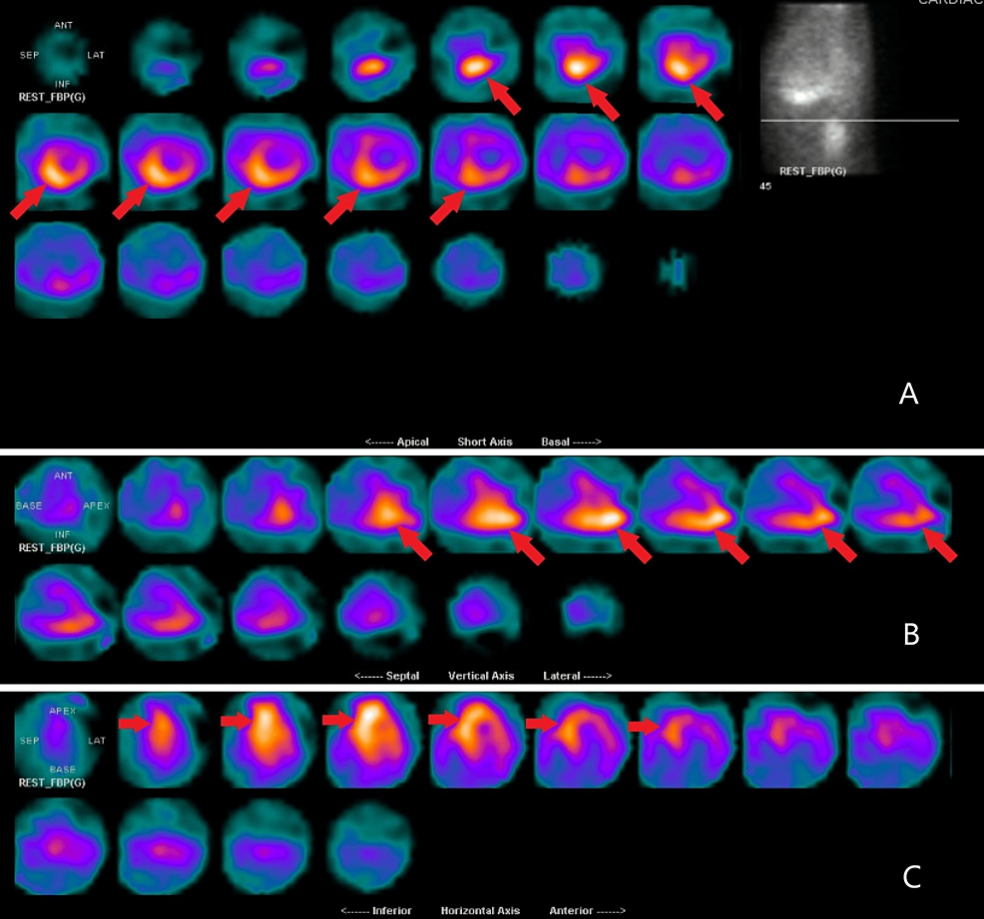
Supine SPECT/CT 99mTc-PYP imaging showing PYP uptake in the left ventricle (A) Short axis, (B) vertical axis, (C) horizontal axis Red Arrows: Focal concentration of 99mTc-PYP in myocardial tissue PYP - pyrophosphate
